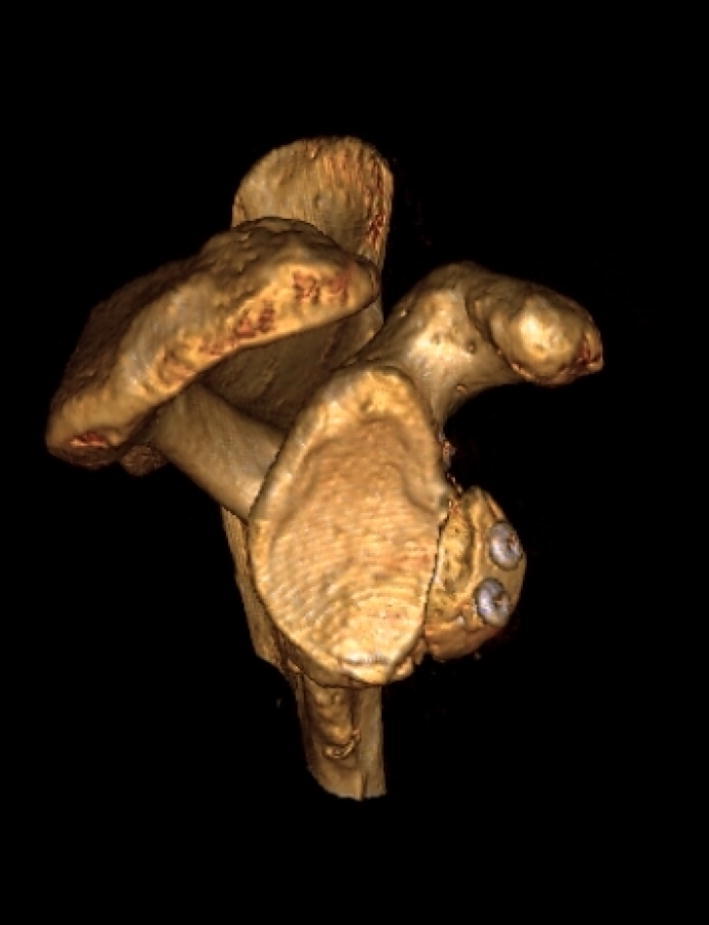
Representative CT image of the bony structures after surgical completion of the sling procedure
